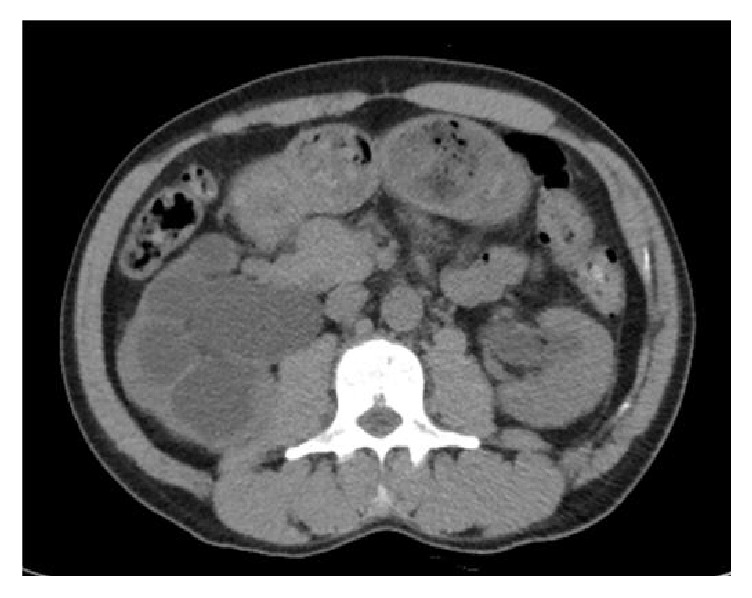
CT scan at the time of admission taken at the level of the kidneys reveals bilateral hydronephrosis, the right greater than the left.
